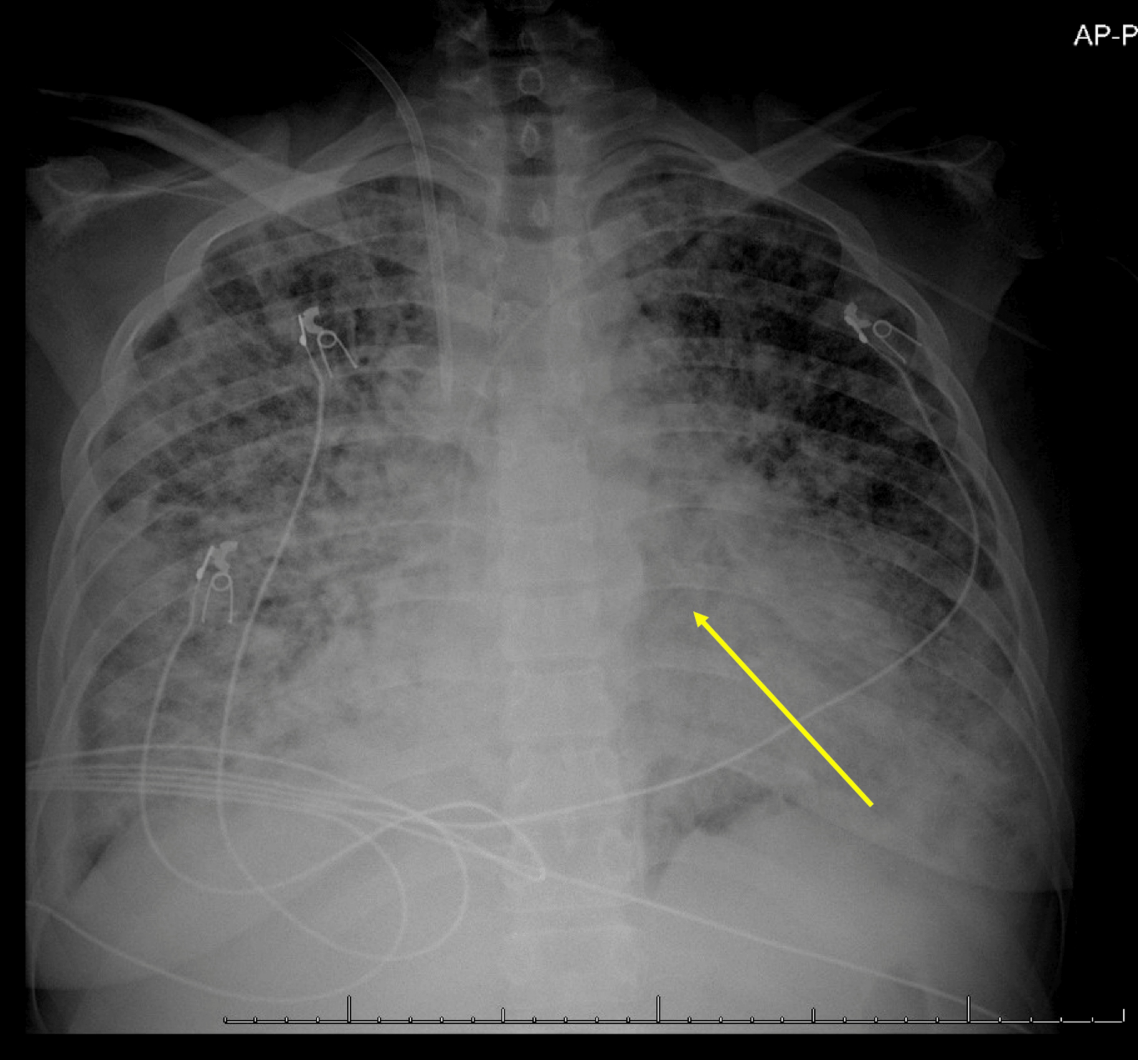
Chest X-ray demonstrating pulmonary edema in sinusoidal obstruction syndrome Anteroposterior (AP) chest X-ray shows diffuse bilateral pulmonary opacities consistent with pulmonary edema (see yellow arrow). Notably, there is increased interstitial marking and alveolar opacities, predominantly in the lower lung fields, suggesting fluid overload. The cardiac silhouette is mildly enlarged, and there is bilateral pleural effusion, more pronounced on the right.
